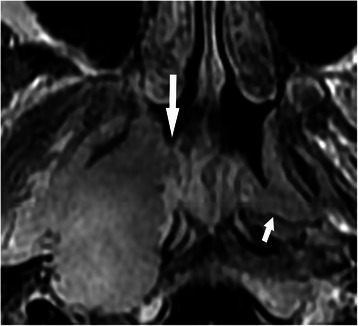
Axial T1-weighted contrast-enhanced MRI before treatment of a 13-year-old boy who had NPC coexisting with hypertrophic adenoids. The interfaces between NPC and hypertrophic adenoids are ill-defined, indicating that the adenoids were invaded (long arrow). The presence of hypertrophic adenoid extension into the left pharyngeal recesses is also detected (short arrow).
